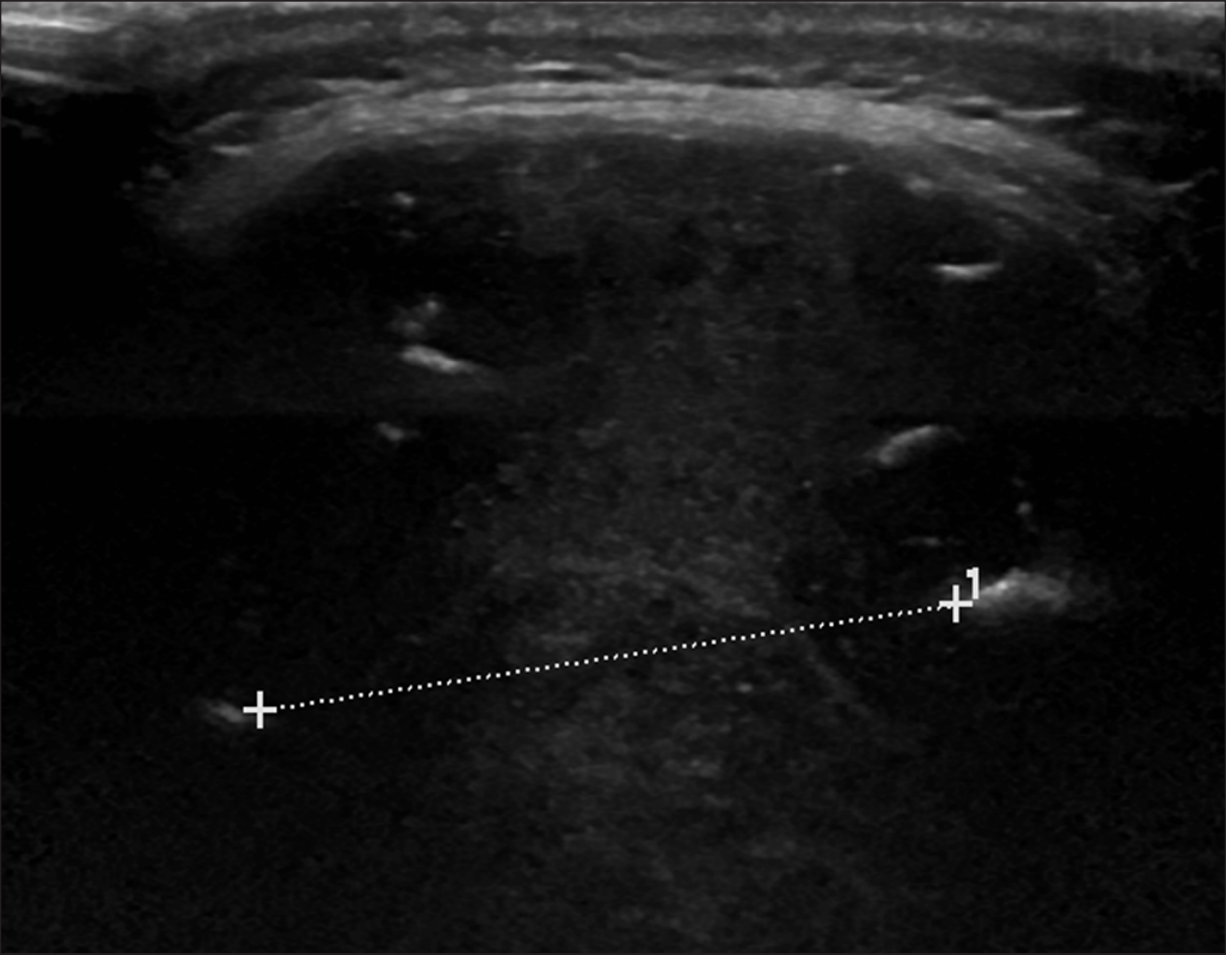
Ultrasound examination of the occipital region shows a heterogeneously hypoechoic mass measuring up to 6 cm.
